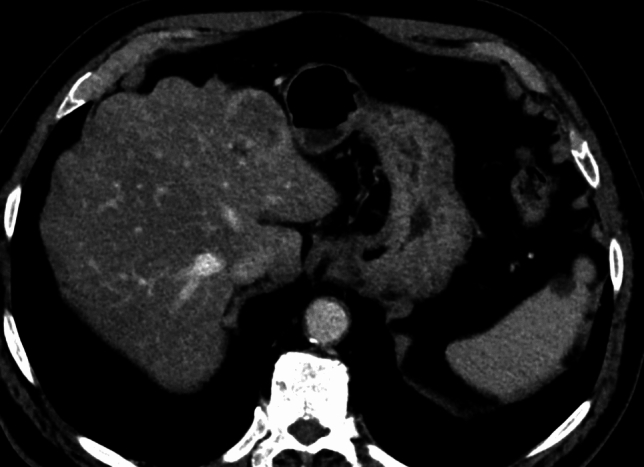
Portal phase Computed Tomography scan representing a liver metastasis surrounded by rim enhancement
